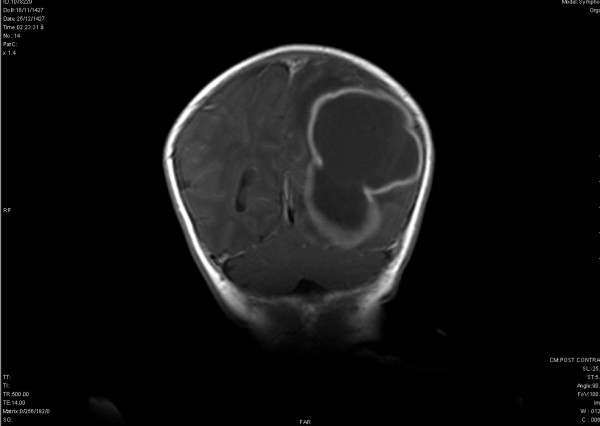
Coronal section of brain MRI with contrast showing a large lobulated collection in the parieto-occipital region.
